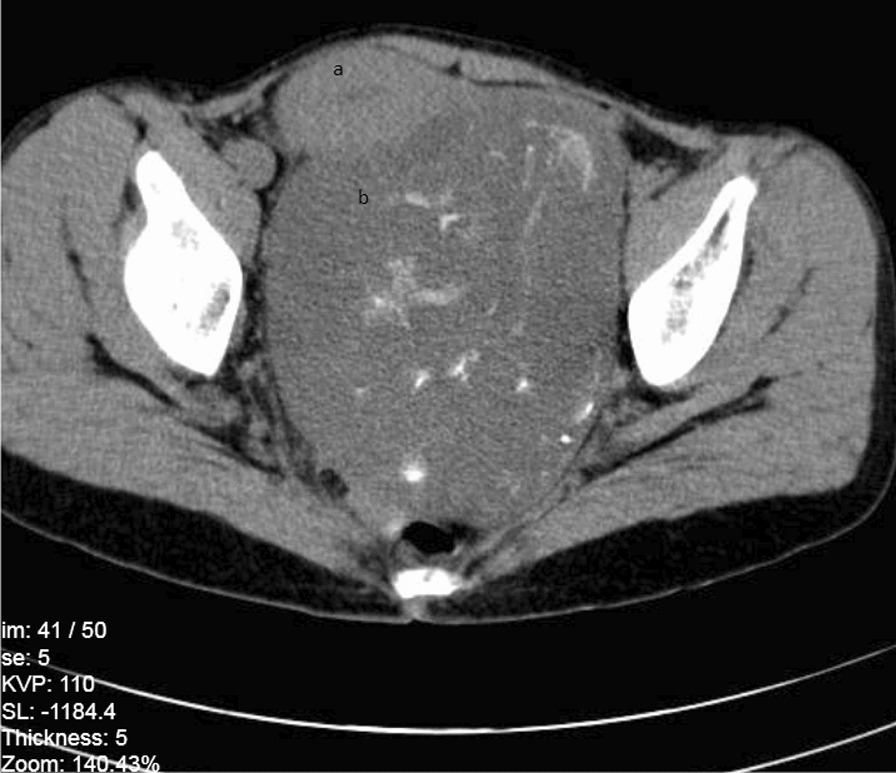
Abdominal CT-Scan without IV contrast, axial view. a Uterine, b Left ovarian mass
